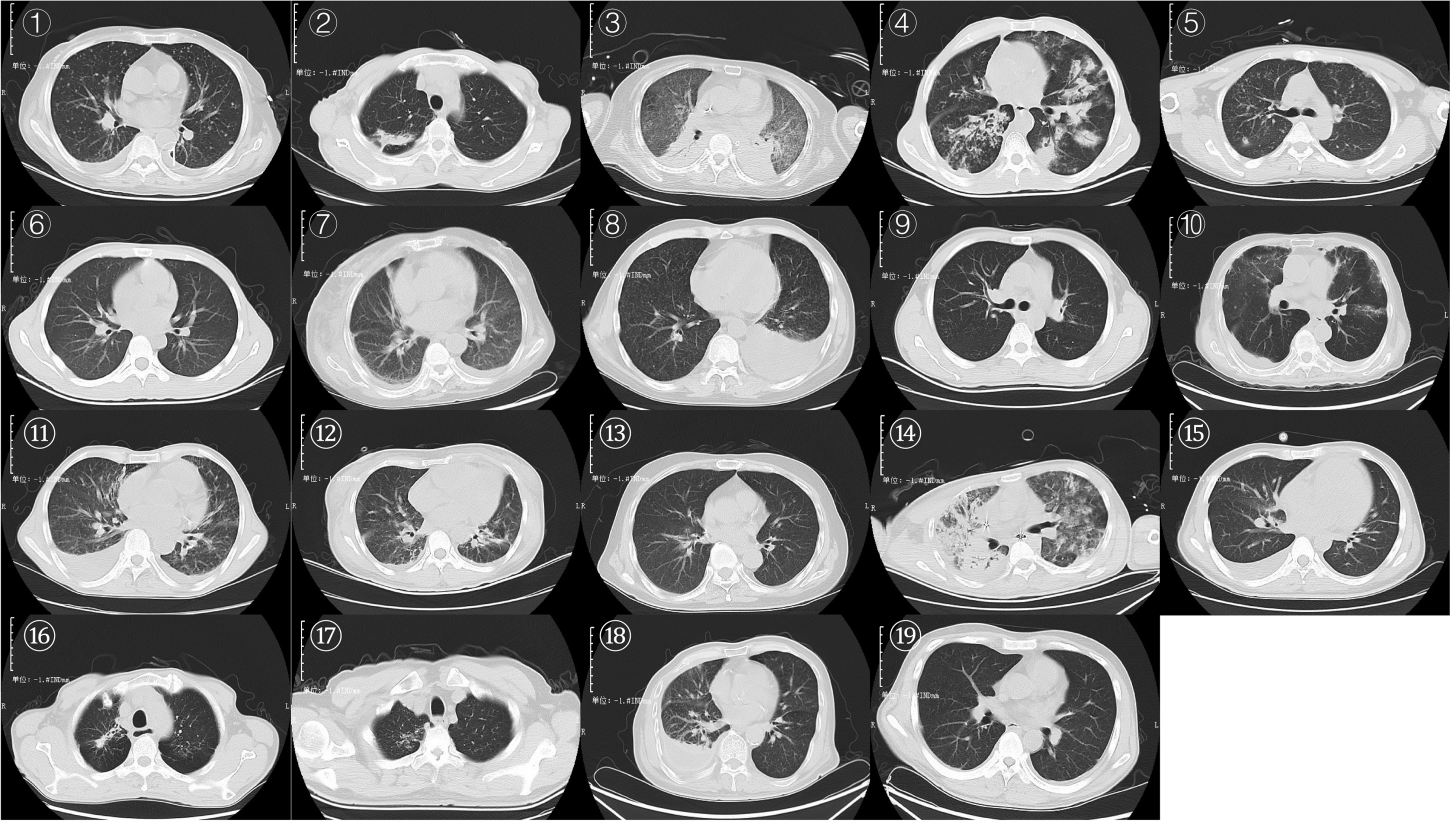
Chest CT imaging examinations of 19 patients.
